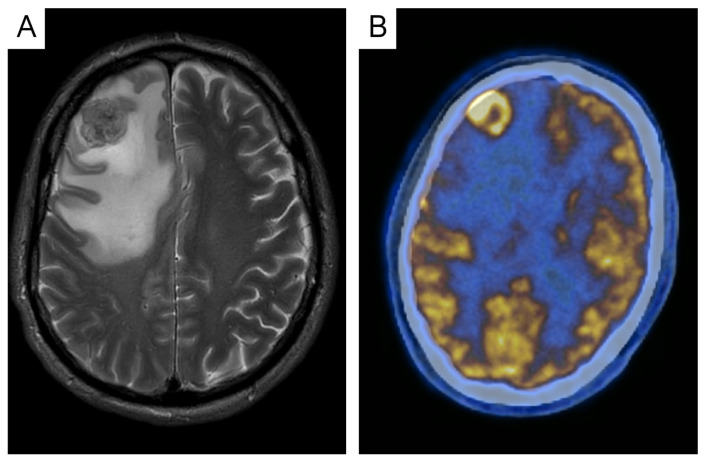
ce-CT vs. PET-CT in the detection of brain metastases (BMs). A large right frontotemporal BM with surrounding edema was clearly diagnosed in the ce-CT head (A), while PET-CT was suggestive of an FDG-avid lesion in this area (B). Abbreviations: ce-CT = contrast-enhanced computed tomography, PET-CT = positron emission computed tomography.
